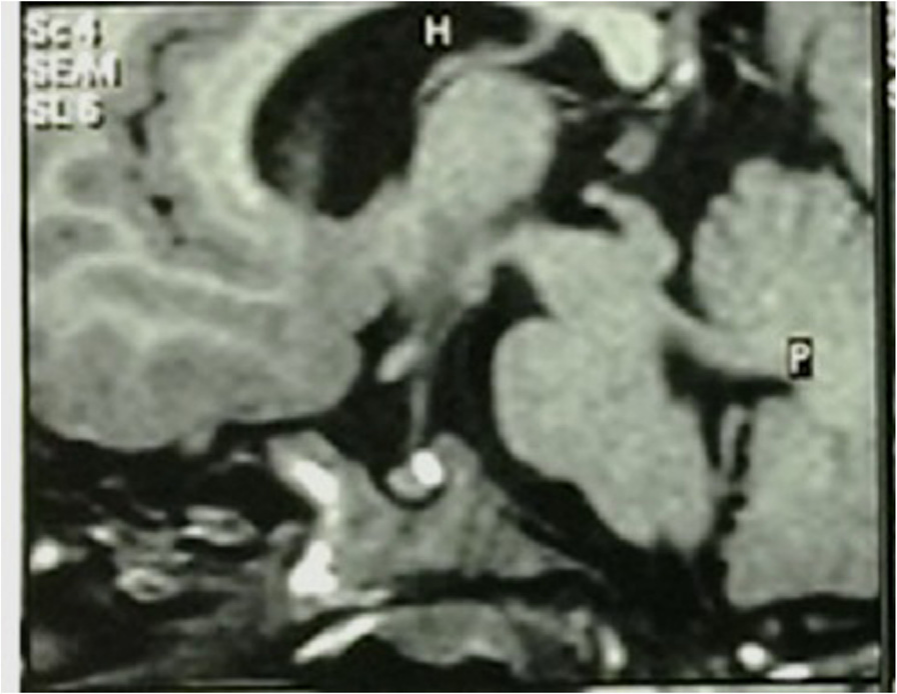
MRI of the brain showing slightly enlarged ventricles and pituitary gland with no abnormalities
